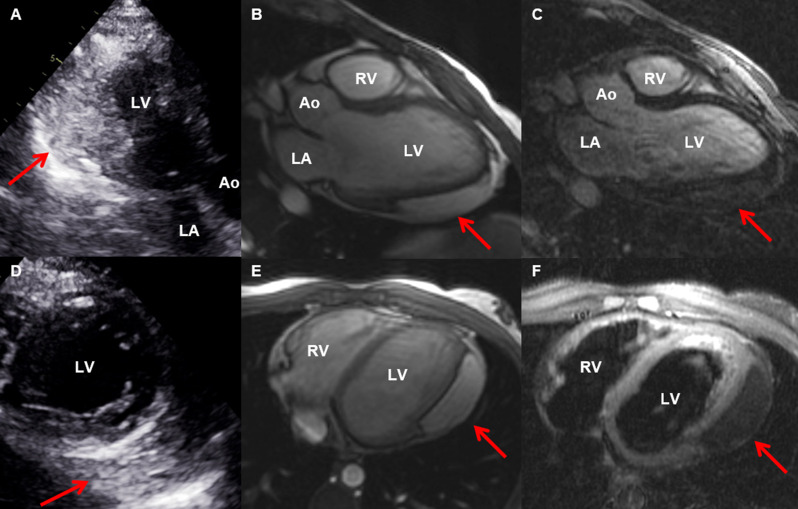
Pericardial lipoma observed via transthoracic echocardiography and cardiac magnetic resonance imaging. Transthoracic echocardiography (A and D) and cardiac magnetic resonance imaging (B, C, E, F) showing a lesion (arrows) in the pericardial region, in close contact with the lateral wall of the left ventricle. In transthoracic echocardiography, a hyperechogenic mass is visualized in the apical three-chamber view (A) and the parasternal short-axis view focused on the left ventricle (D). Cardiac magnetic resonance imaging revealed a lesion with an adipose component in the three-chamber view (B and C) and the longitudinal axis of the ventricles (E and F). No late contrast enhancement was observed (C). In F, a hypointense signal is noted in the T2-weighted fat-saturation sequence, suggesting a lesion with a fatty component. LA: left atrium; RV: right ventricle; LV: left ventricle; Ao: aorta. Exams performed at the Heart Institute
